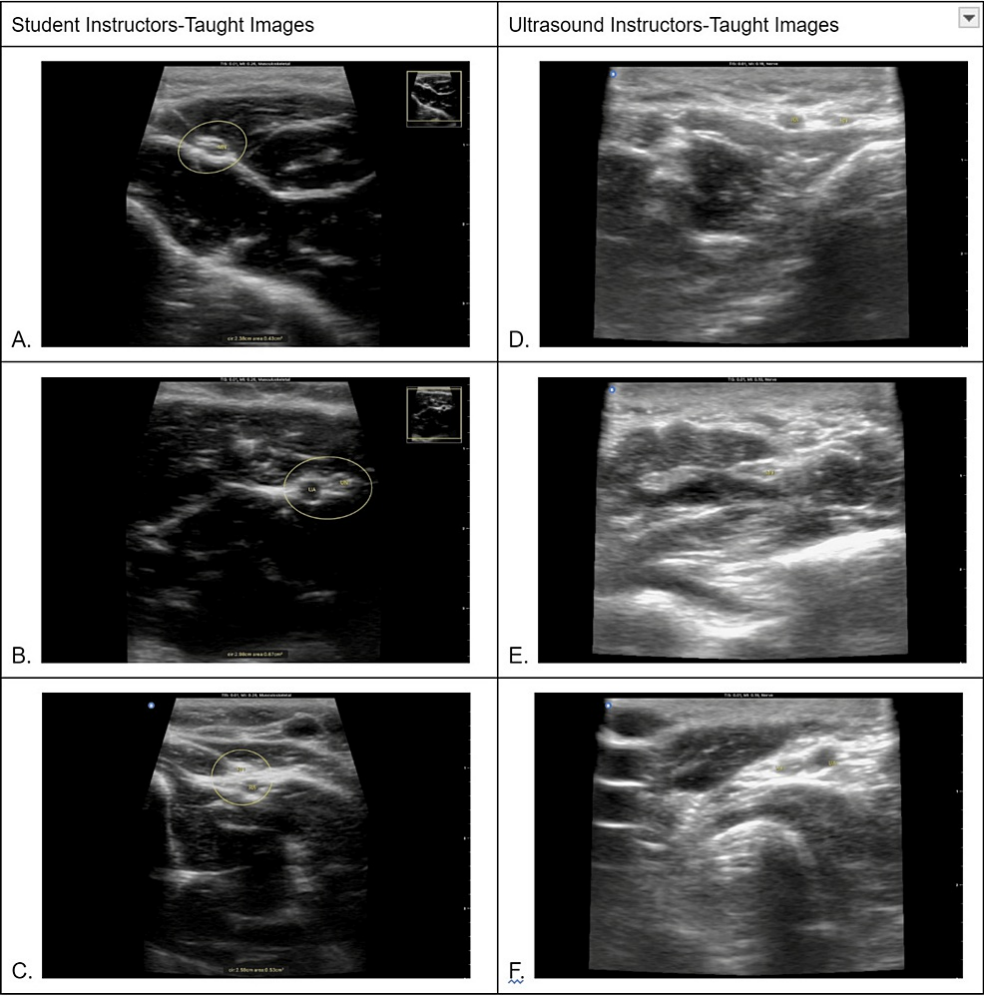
Side-by-side comparison of correctly labeled student ultrasound images between teaching groups. Images A, B, and C are images captured by students taught by STs and identify the median nerve, ulnar nerve, and artery, and radial nerve, respectively. Images D, E, and F were captured by students taught by UIs and identify the median nerve, ulnar nerve and artery, and radial nerve, respectively.
